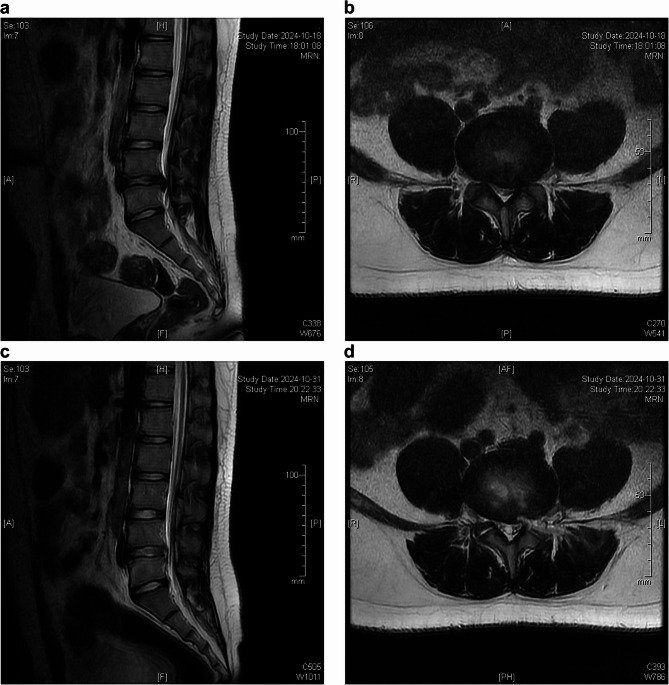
A male patient with lumbar disc herniation at L4,5 underwent navigated PELD surgery (a)(b) preoperative images of MRI scan (c)(d) postoperative images of MRI scan
